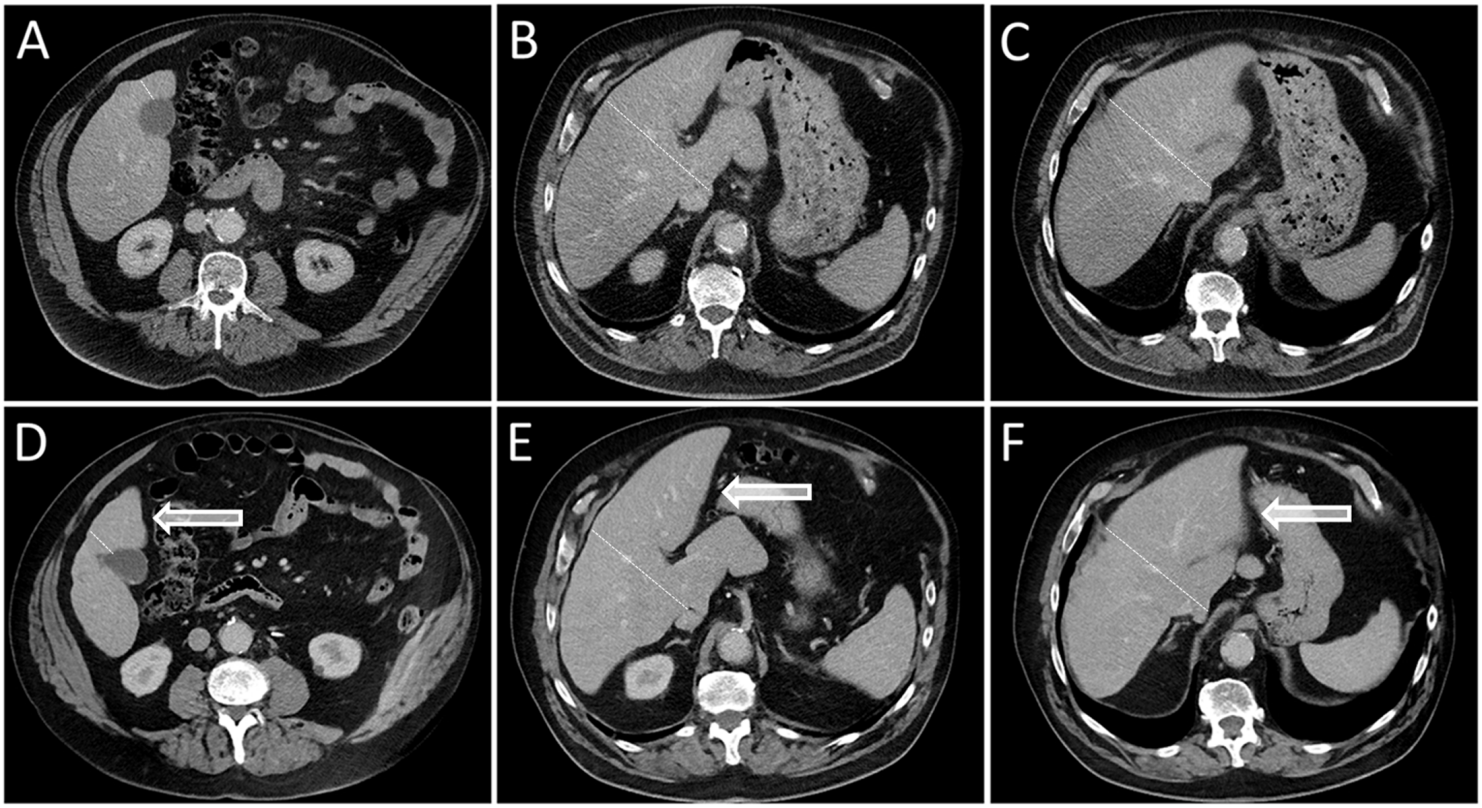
Example of left lobar hypertrophy after right-sided RE. Liver computed tomography images acquired in three different levels before RE (A-C) and 6 months after right-sided RE (D-F) show left lobar hypertrophy (highlighted by arrows) and right lobar atrophy in a 65-year-old man suffering from HCC and non-alcoholic steatohepatitis. (The liver lobe border is marked by a dotted line.).
